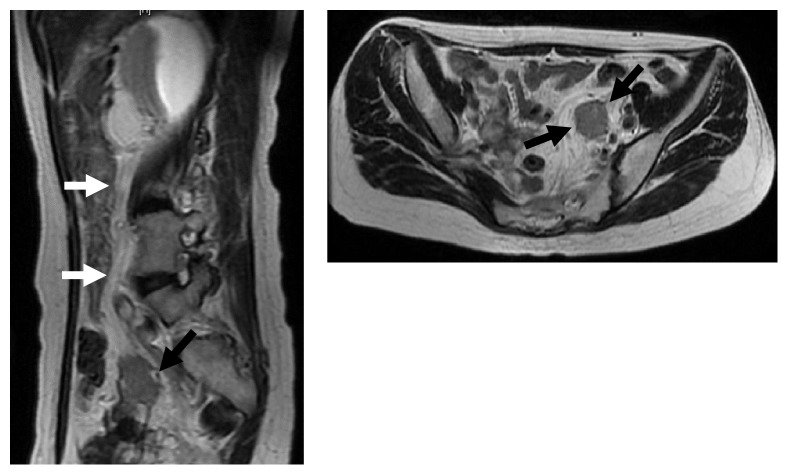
MRI findings of (a) the abdomen and (b) the pelvis with T2-weighted images: white arrows: left ureter and black arrows: a postperitoneal tumor.
